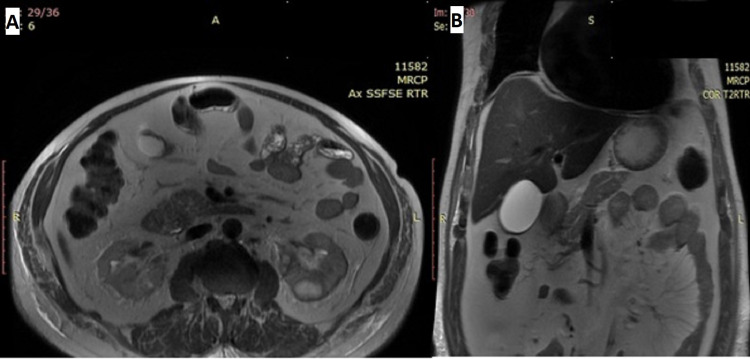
On magnetic resonance cholangiopancreatography, bilateral kidneys show irregular margins with perinephric T2/STIR hyperintense signal. No features suggestive of pancreatitis. (A) Axial section (there is a T1 hypointense cortical cyst in the left kidney at the lower pole), (B) Coronal section. T2/STIR: T2-weighted short-tau inversion recovery.
